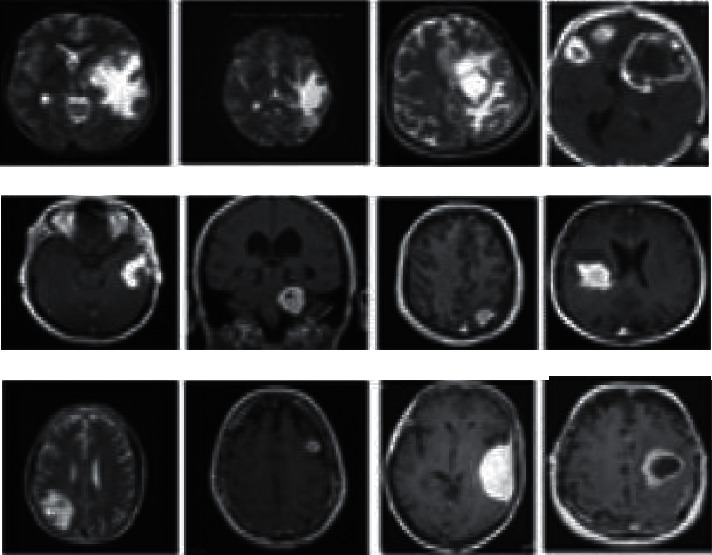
3D brain tumor MRI images of the REMBRANDT dataset.
